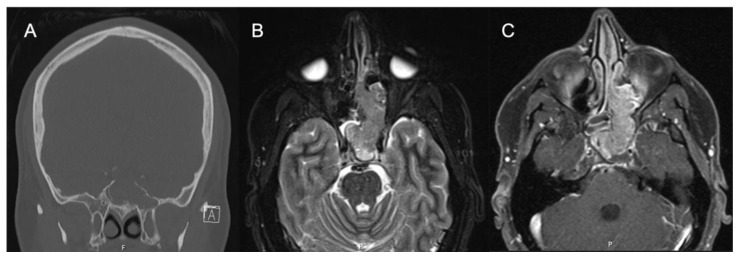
IP-degenerated squamous cell carcinoma. (A) Non-contrasted CT sinus demonstrating a mass of the left sphenoid with erosion of the skull base at the sella. (B) STIR sequence contrasted MRI demonstrating an expansile mass originating from the left sphenoid lateral wall. (C) T1 sequence contrasted MRI with fat suppression demonstrating a mass of the left sphenoid and ethmoid cavity.
